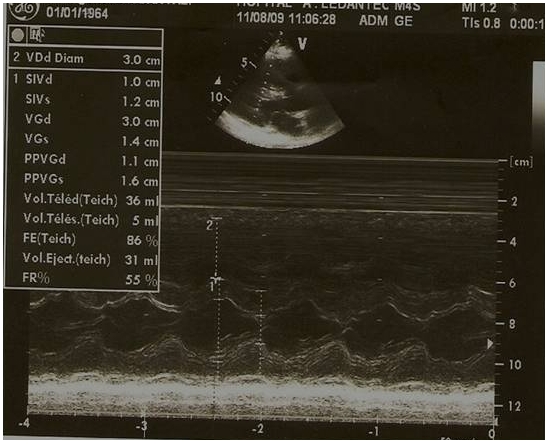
Image d’échocardiographie mode TM, coupe parasternale grand axe, incidence transventriculaire montrant une dilatation modérée du ventricule droit
